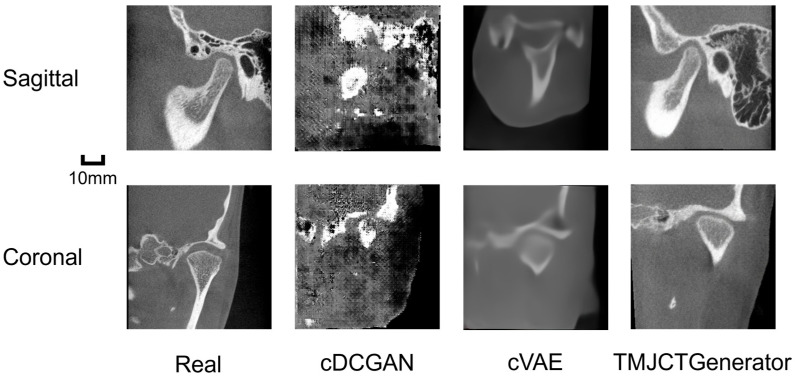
Visual comparison of real and synthesized TMJ CBCT images. The rows display representative samples in sagittal and coronal views. The columns compare real patient scans with images generated by the baseline conditional VAE (cVAE), conditional DCGAN (cDCGAN) and the proposed TMJCTGenerator. All generated samples shown were randomly drawn without quality-based selection.
